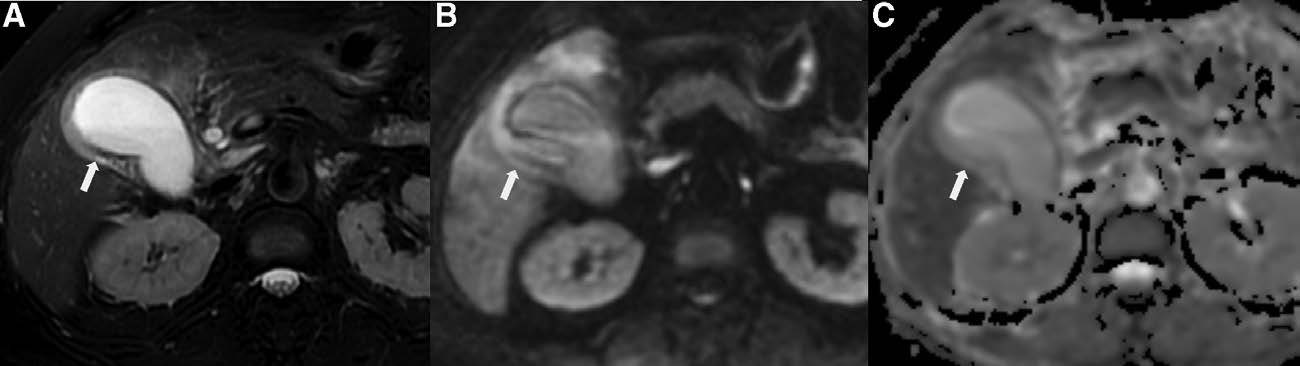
Acute onset of chronic cholecystitis in a 61-year-old female. (a)Diffuse gallbladder wall thickening (arrow) with layered appearance was exhibited on T2WI with fat saturation image (type 2); Hypointensity was observed in the inner wall of GB and hyperintensity in the outer wall, without papillary change. (b) Diffuse hyperintensity with layered appearance on DWI image was shown (arrow). (c)The layered pattern (arrow) was shown more clearly on the ADC map image (type 2). DWI = diffusion-weighted imaging, T2WI = T2-wighted imaging.
